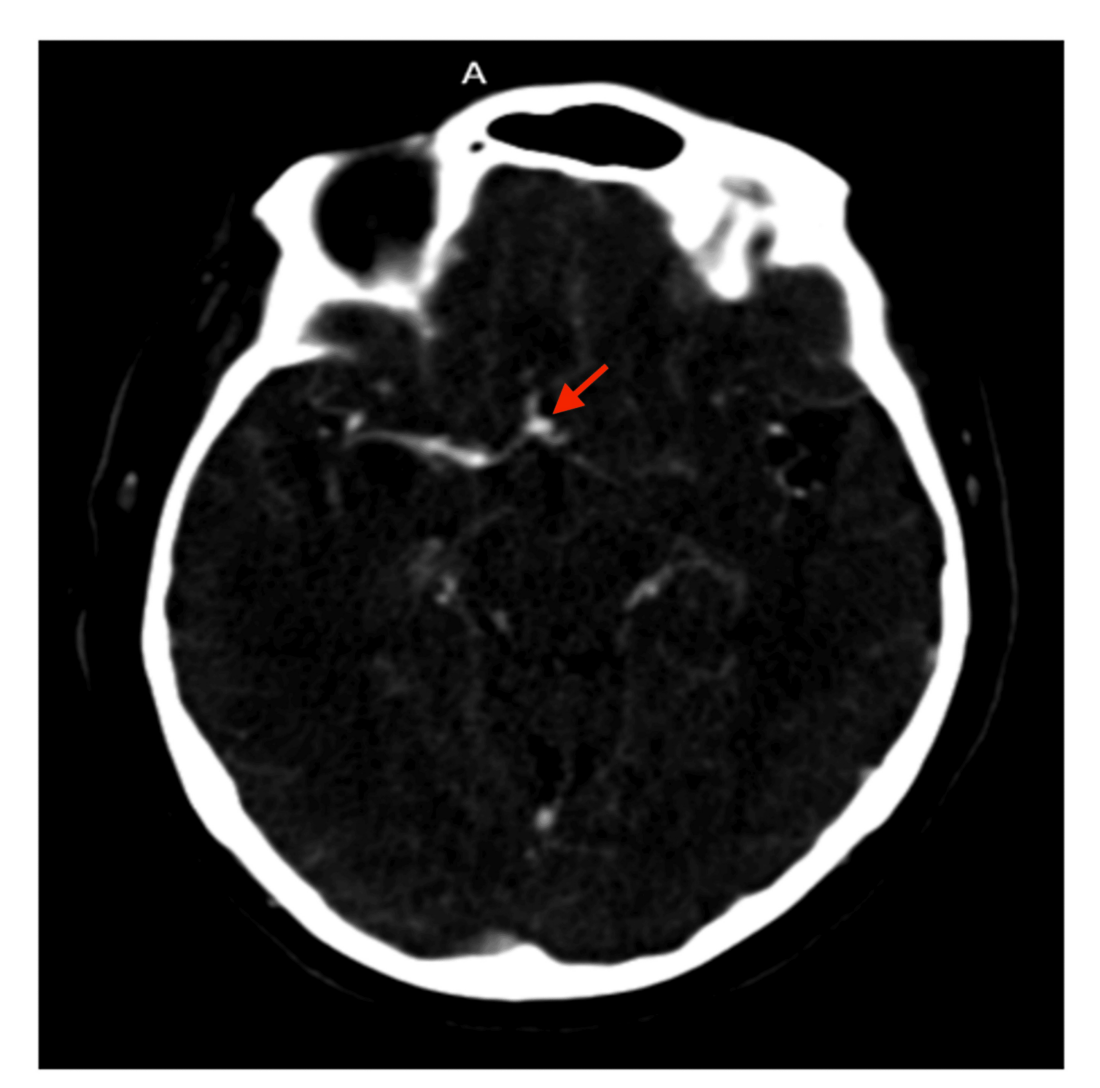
Dilatation of the proximal segment of the ACA, measuring 4 mm and suggestive of a suspected aneurysm in the right ACA or at the ACOM, along with irregularity in the right MCA, as seen on axial arterial-phase CTA of the brain (red arrow). ACA, anterior cerebral artery; ACOM, anterior communicating artery; MCA, middle cerebral artery; CTA, computed tomography angiography
